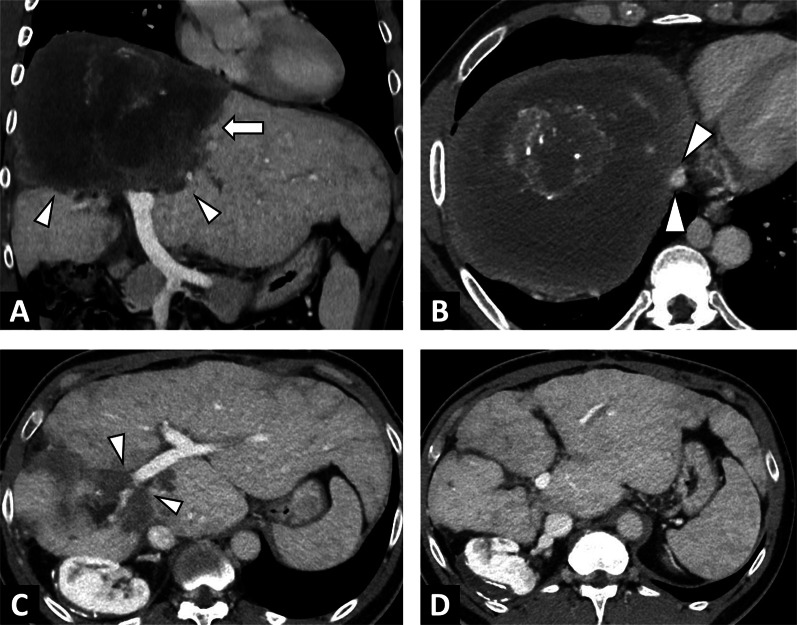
Alveolar echinococcosis lesion of the right liver in a 44-year-old man complicated by advanced chronic liver disease secondary to Budd Chiari syndrome. A CT image in the coronal plane at the portal phase showing alveolar echinococcosis of the right liver (arrow) associated with infiltration to the pedicle (arrowheads). B CT image in the axial plane obtained during the portal phase: invasion of the inferior vena cava and the ostium of the hepatic veins (arrowheads). C CT image in the axial plane obtained during the portal phase: infiltration of the right liver pedicle (arrowheads) D. Hypertrophy of the left liver lobe and surface nodularity suggestive of advanced fibrosis. Note the mosaic pattern of enhancement, sign of hepatic sinusoidal dilatation, as a result of the Budd Chari syndrome
